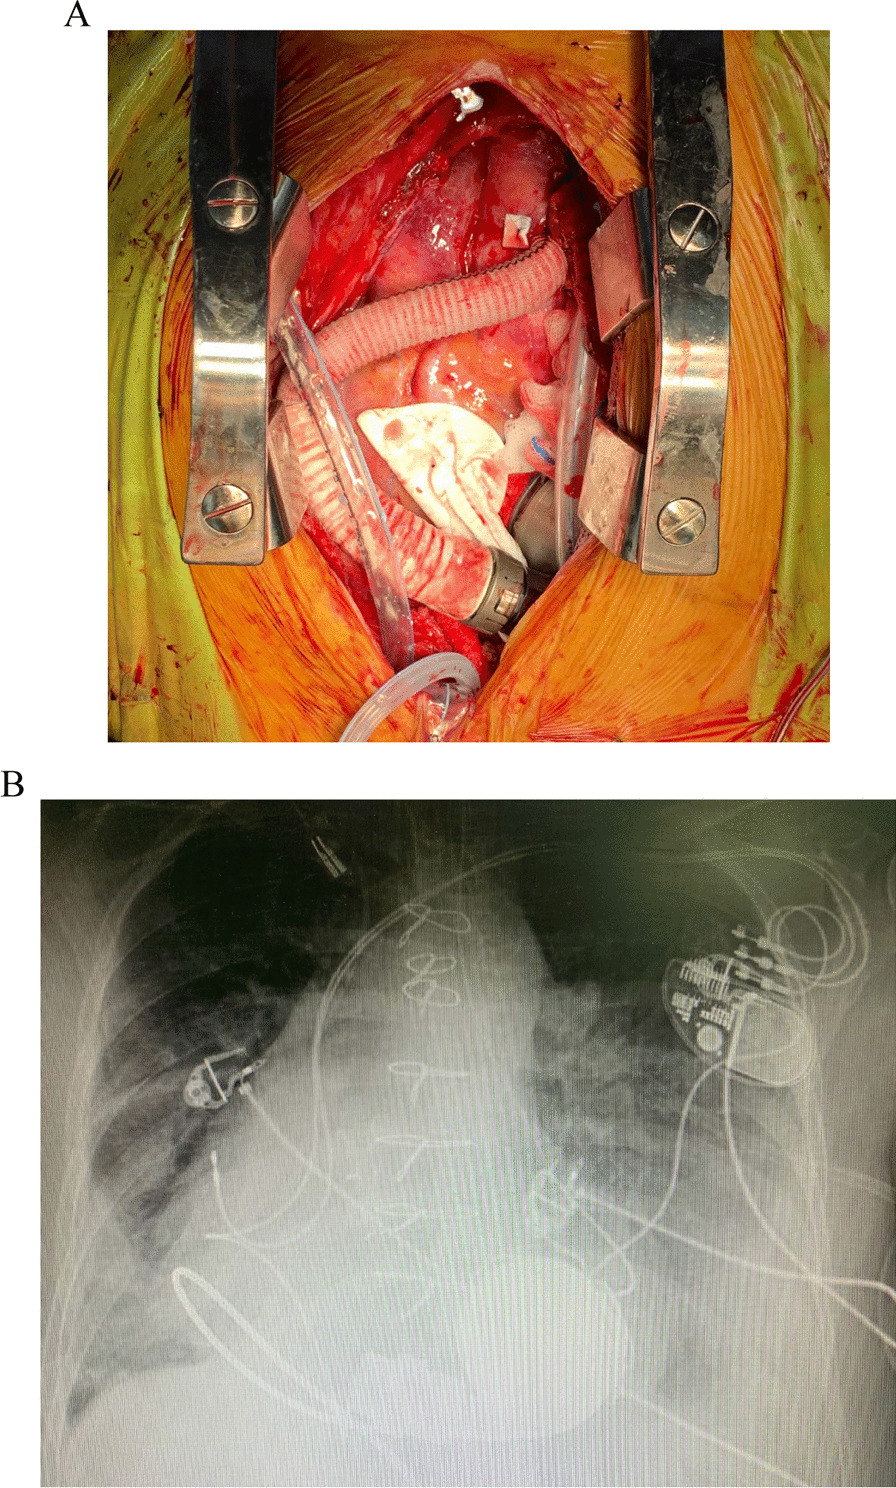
A HM3 implanted in the heart of the ccTGA patient, the graft pathway in the chest is unique and longer as the aorta is located left to the PA. B Chest X-Ray demonstrating the direction of the inflow cannula in the heart of the ccTGA patient directed anterior to posterior
